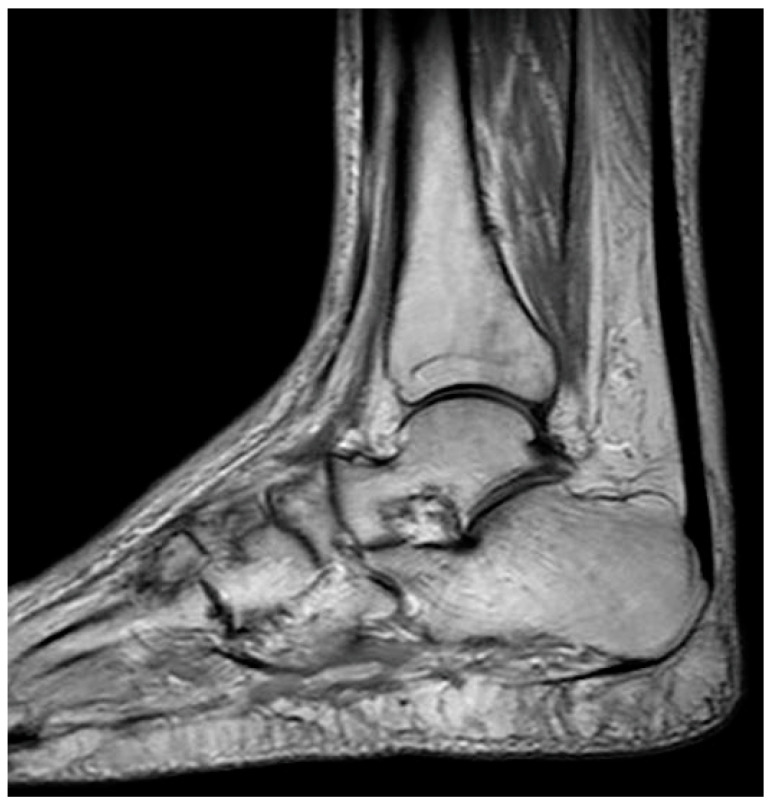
T1-weighted image of AVN of the talus bone.
